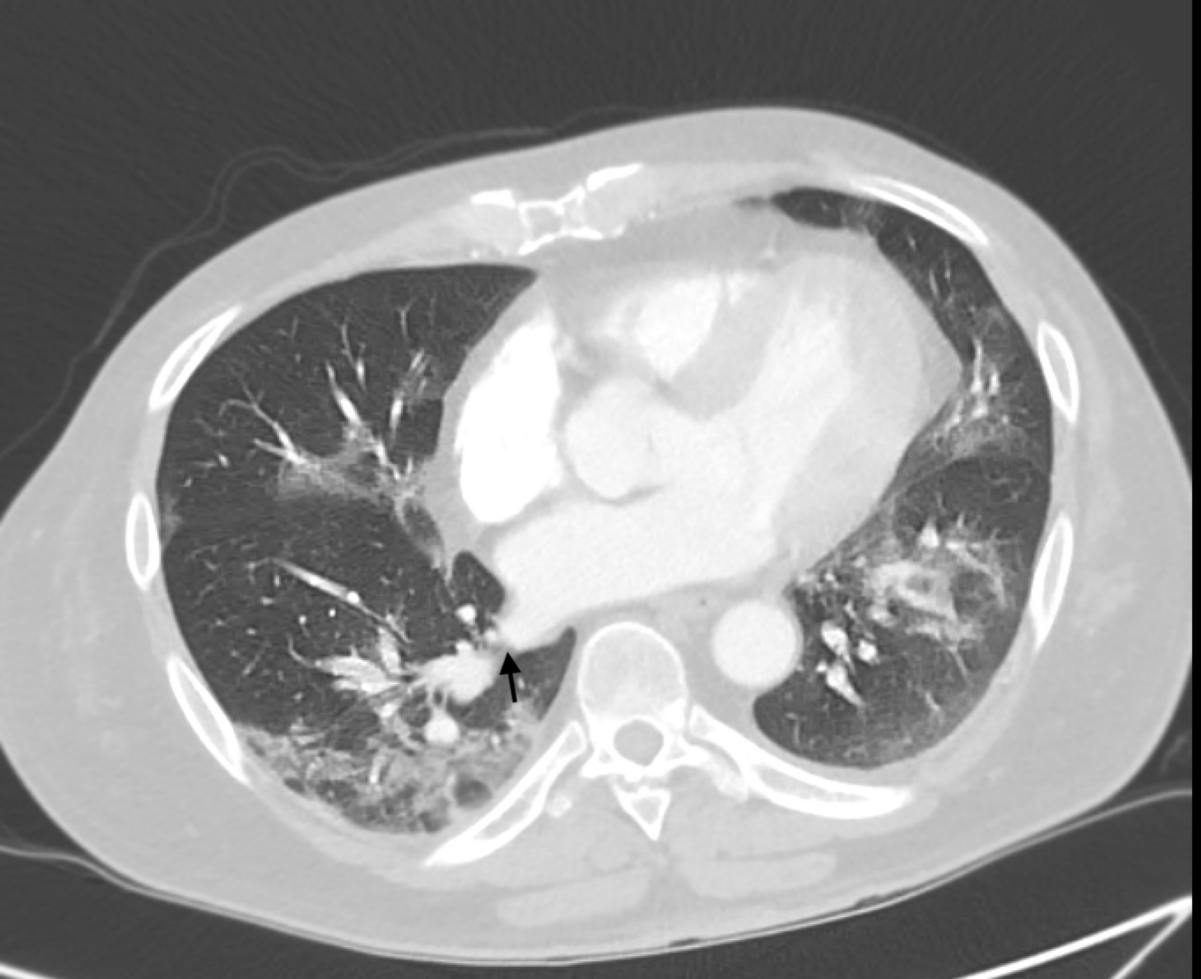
CT angiography of the chest revealing pulmonary emboli in the right lower lobe.
